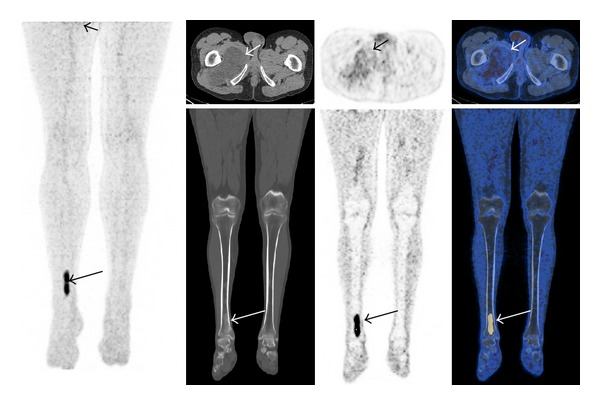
False positive 48 year old with a large myxoid liposarcoma of the right thigh (short arrow) with low metabolic activity (SUV 2.6) required biopsy to demonstrate that an avid (SUV 7.4) lesion of the right distal tibia (long arrow) was fibrous dysplasia.
